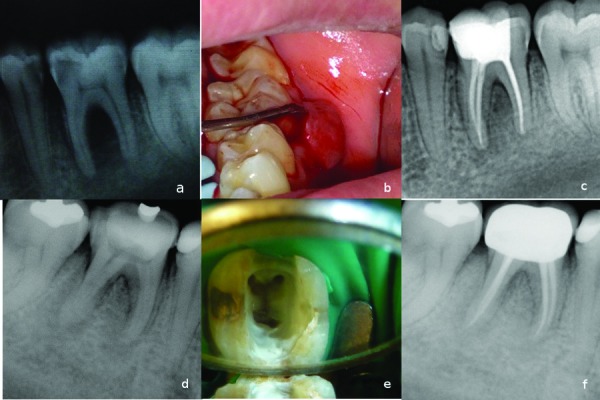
Case 1- a) Initial radiograph of tooth #36. b) Intraoral film showing increased probing depth values in the furcation area. c) A year recall radiograph showing complete healing. Case 2- d) Initial radiograph showing increased bone lesion in the furcation and distal side of the tooth #46. e) Access opening showing mesio-distal crack line in the pulp floor. f) A year recall radiograph showing healing of the bone lesion.
